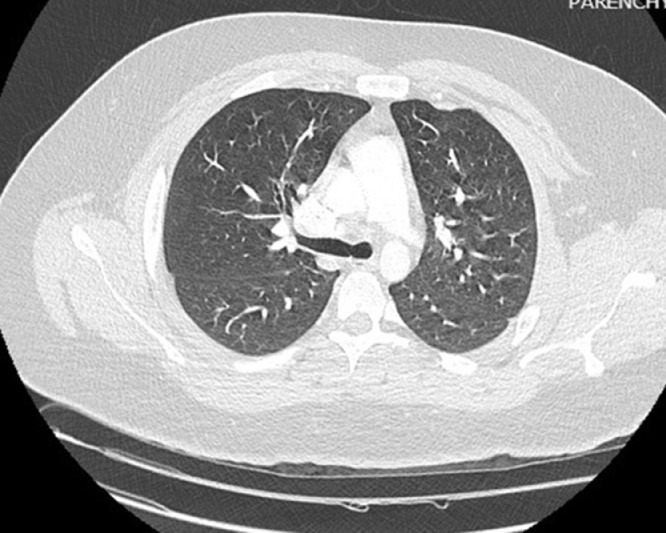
Chest CT on admission: normal lung parenchyma.
